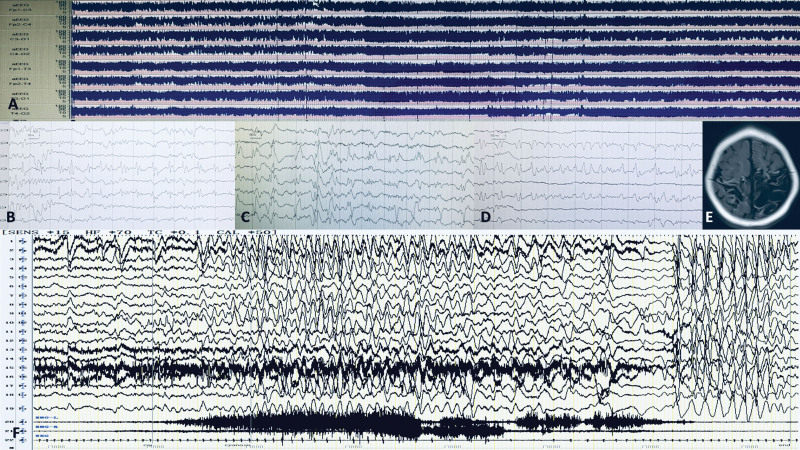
Case 6 female. (A to D) Bedside cEEG at 29 d age: (A) aEEG showed sustained state of focal seizures (with a large amount of arched elevation at the lower boundary). (B, C, and D) 3 times focal seizures respectively: EEG showed rhythmic discharge starting from the left or right brain area. (E) head MRI showed cephalomalacia. (F) VEEG at 1 yr 5 mo age: slow-flat-low patterns with BHS. aEEG = amplitude e integrated EEG, BHS = breath-holding spells, cEEG = continuous EEG, MRI = magnetic resonance imaging, VEEG = video electroencephalogram.
